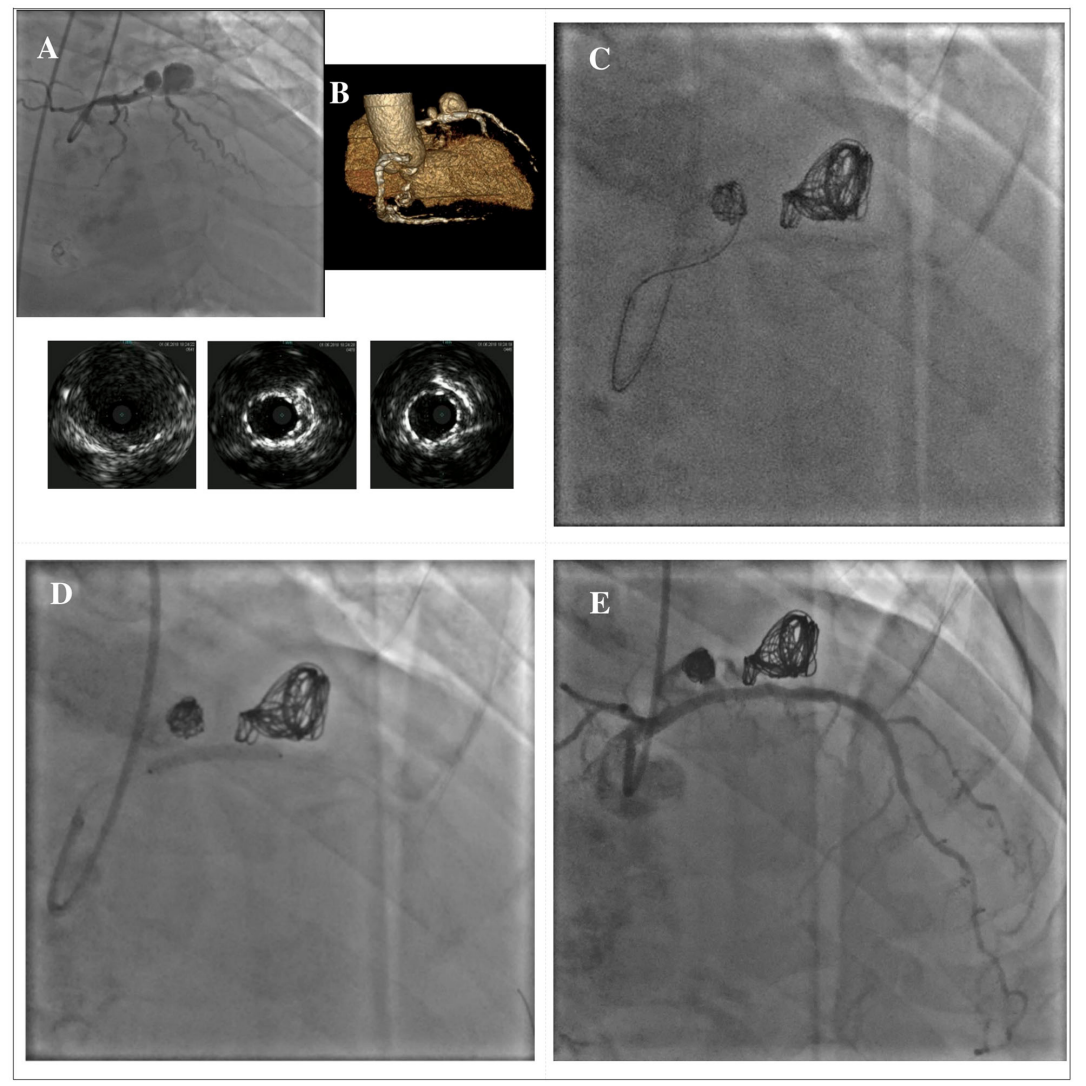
Hybrid approach for combined treatment with stent-assisted coil embolization and covered stent implantation in a dual-chamber iatrogenic pseudoaneurysm following PCI of the proximal LAD. The upper panel shows the angiographical view of an iatrogenic pseudoaneurysm of the proximal LAD due to a previous PCI with DES implantation; the lower panel shows the IVUS images of the pseudoaneurysm and its relationship with the previously implanted DES (A). Rendered volume CCTA image showing a large dual-chamber pseudoaneurysm (B). Multiple coils are advanced through a microcatheter to wrap around the old DES until completely filling both cavities of the pseudoaneurysm (C). A BeGraft covered stent (Bentley InnoMed, Hechingen, Germany) 3 x 24 mm has been used to secure the coils correct positioning (D). Final result (E). ^*^Courtesy of Dr. Fabio Felice Tarantino. CCTA, coronary computed tomography angiography; DES, drug-eluting stent; IVUS, intravascular ultrasound; LAD, left anterior descending; PCI, percutaneous coronary intervention.
